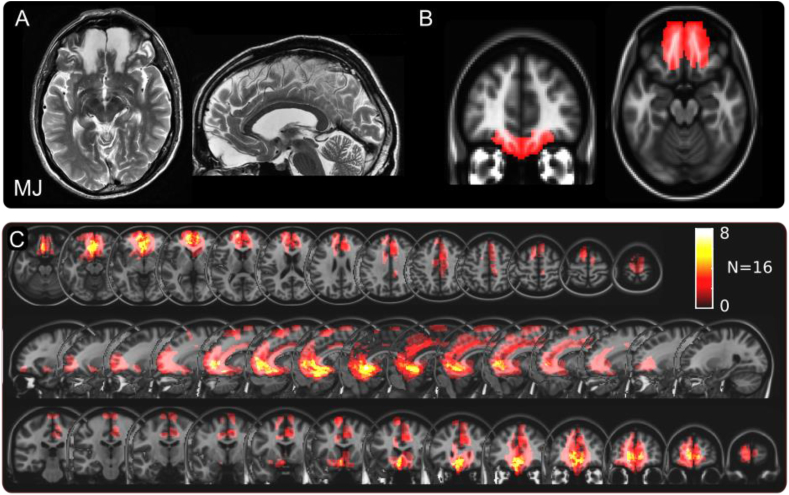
Medial prefrontal cortical (PFC) lesions. A Representative slices from T2-weighted MRI scan showing patient MJ's lesions, showing bilateral damage involving vmPFC/medial OFC. B MJ's lesions mapped onto MNI template. C Overlap map of lesions for the 16 patients with unilateral medial PFC damage.
